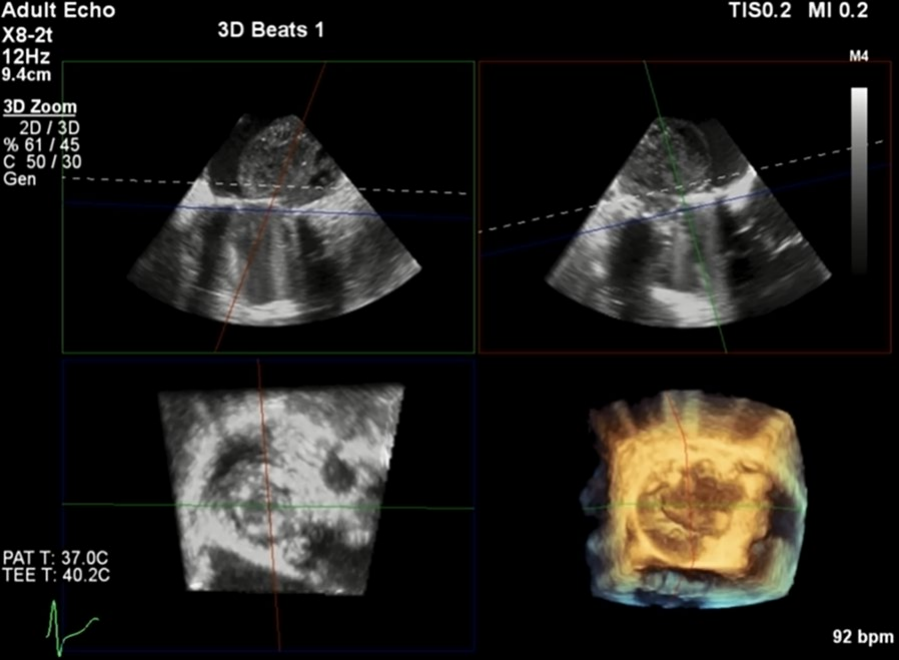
Large non-homogenous fixed mass (4 × 4 cm) on lateral left atrial wall with restricted mitral valve motion (mid-esophageal view and three-dimensional image in transesophageal echocardiography)
